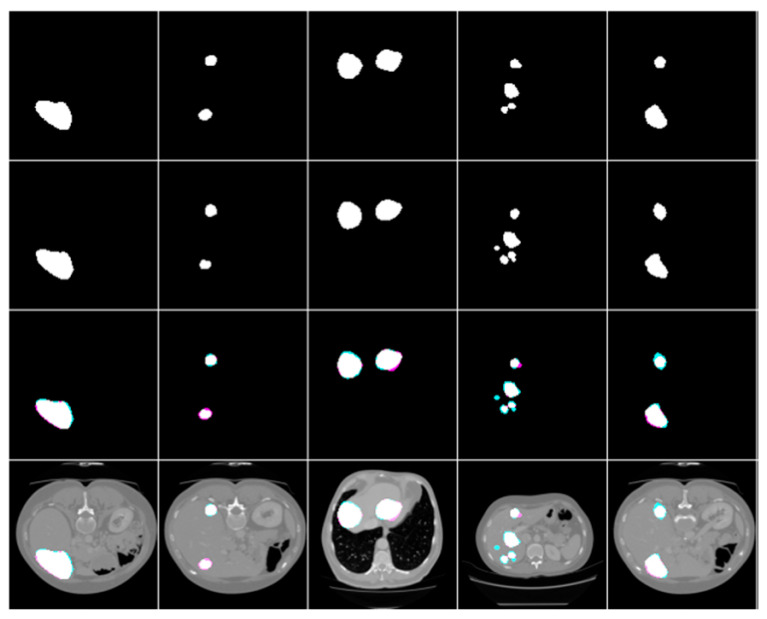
The end result of using masks and overlap pictures to segment a tumor from abdominal CT scans.
